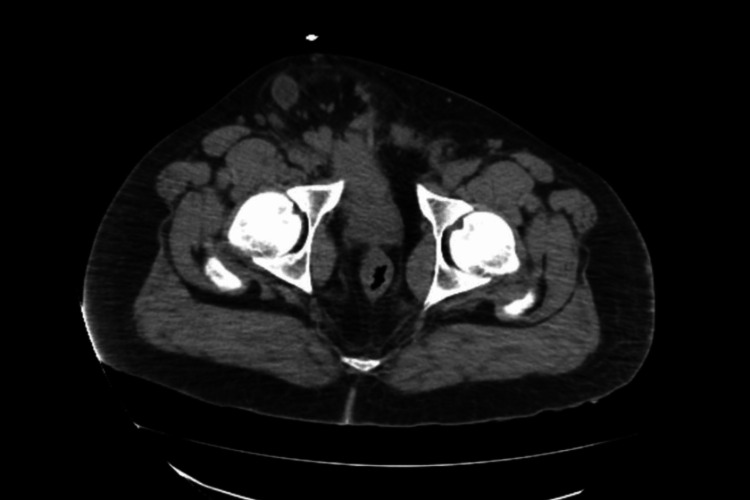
Axial view on CT demonstrating distal ureteric involvement into the scrotum.
